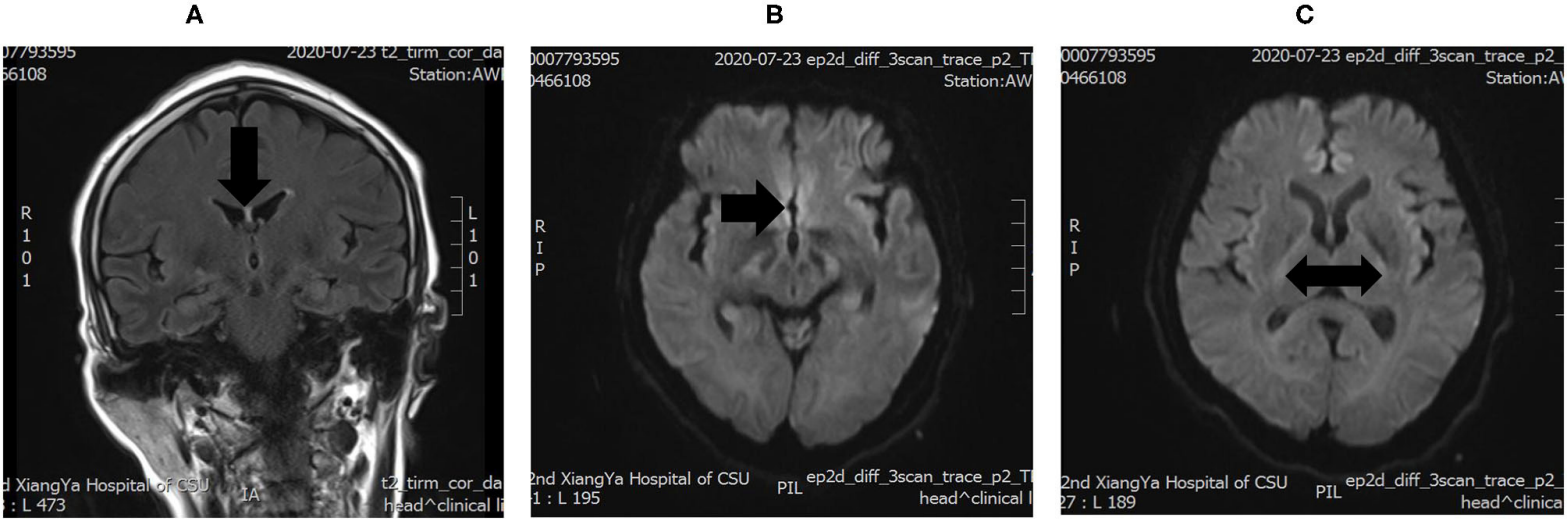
(A) increased T2 signals in the periventricular region around the third ventricle. (B,C) Diffusion-weighted imaging (DWI) showing Wernicke's encephalopathy (WE) changes in the bilateral basal ganglia.
